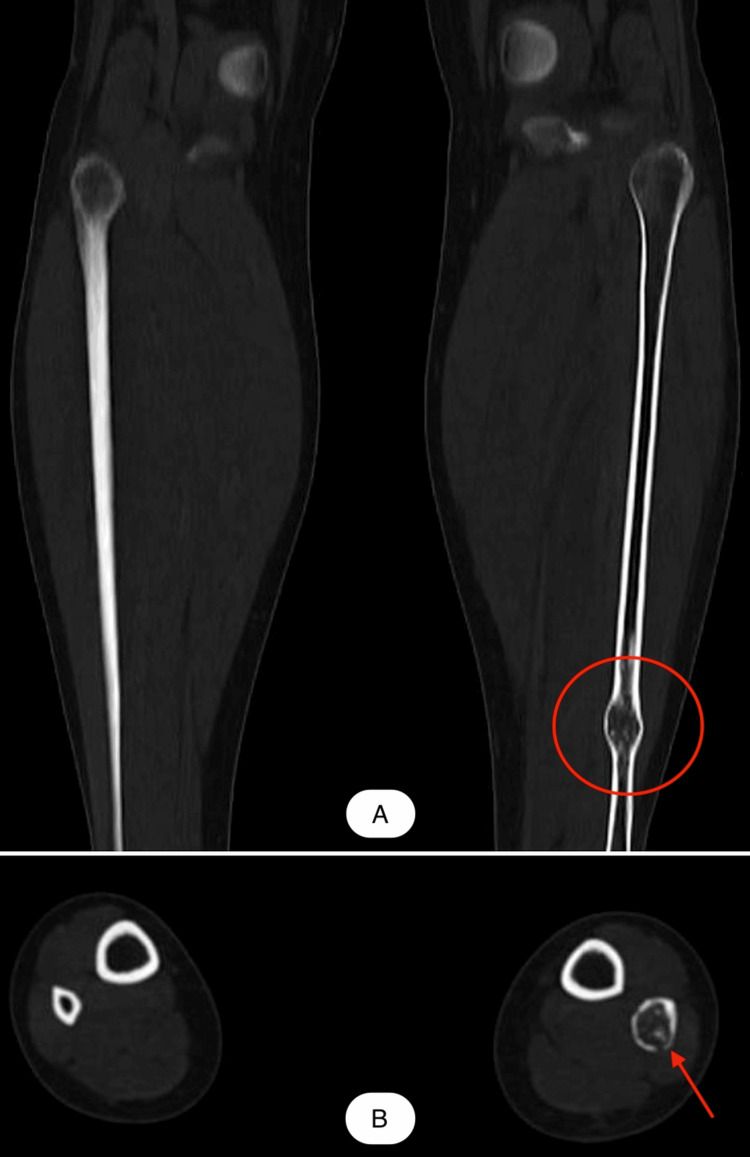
Computed tomography (CT) scan distal 1/3 of the fibula shaft before surgery A - coronal view B - axial view
